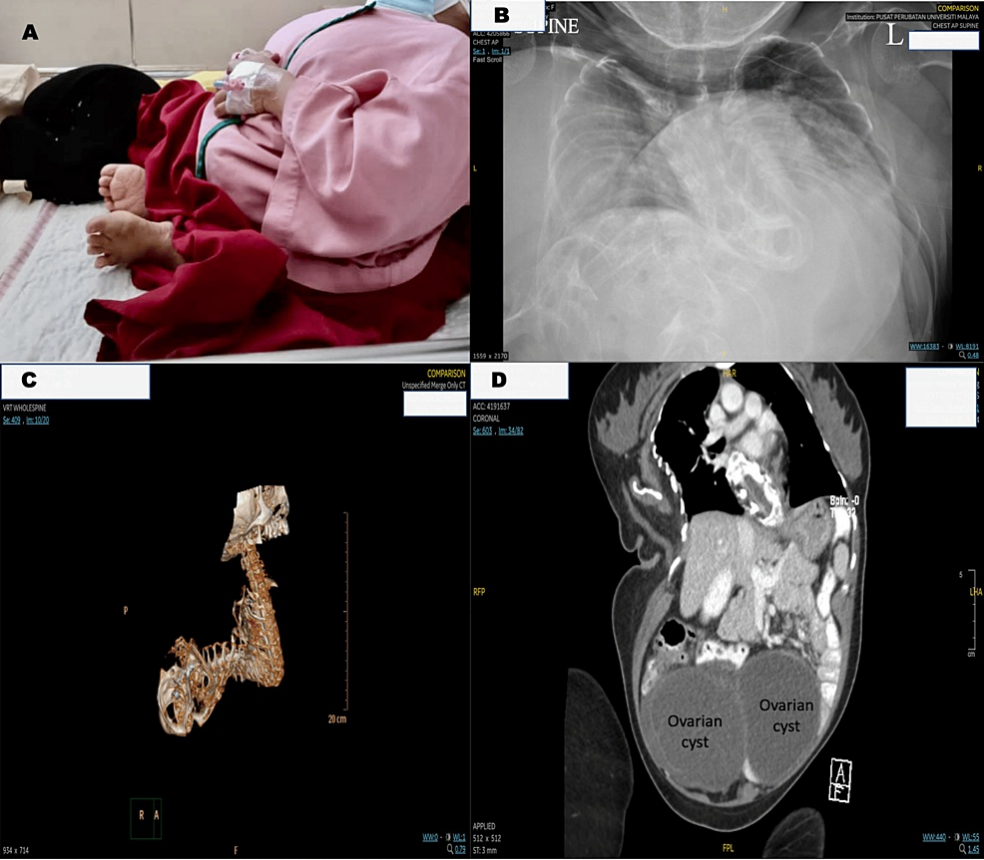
Patient's background medical problems A. The patient’s short stature and severe physical disabilities resulted from osteogenesis imperfecta. She was largely wheelchair-bound and semi-independent in the activities of daily living. B. Chest X-ray showed very poor lung reserve. Her lung function test showed severe restrictive lung disease (forced expiratory volume in 1 second, FEV1 0.36L; forced vital capacity, FVC 0.44L; FEV1/FVC 81%). C. Computed tomography (CT) of the whole spine showed severe kyphoscoliosis. Multiple fractures of varying ages were reported to be present in bilateral first ribs, the costovertebral junction from T2 to T5 levels on the right, T2 to T4 levels on the left, and the bilateral femoral neck. CT thorax, abdomen, and pelvis showed bilateral large multi-loculated ovarian cysts (right side: 12.1 x 9.3 x 10.3cm; left side: 6.8 x 9 x 7cm)
